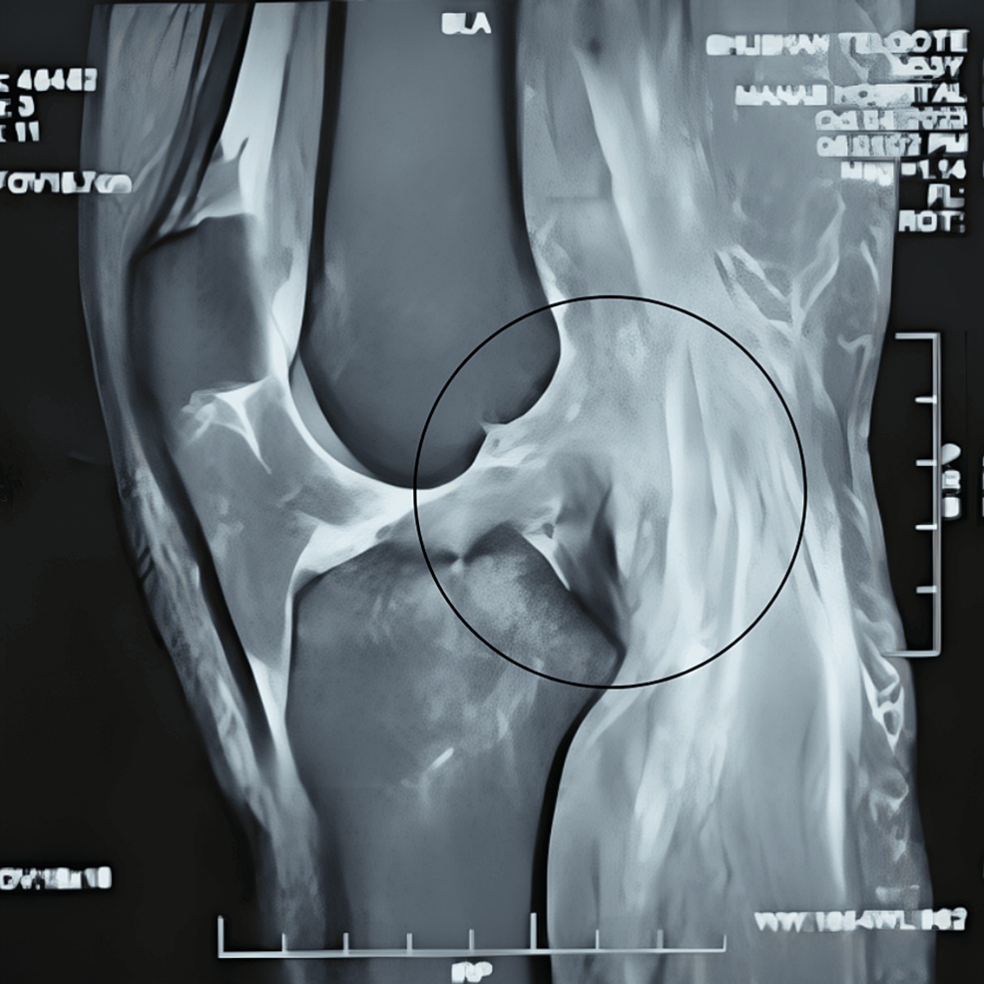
MRI showing PCL tear MRI: Magnetic resonance imaging; PCL: Posterior cruciate ligament
